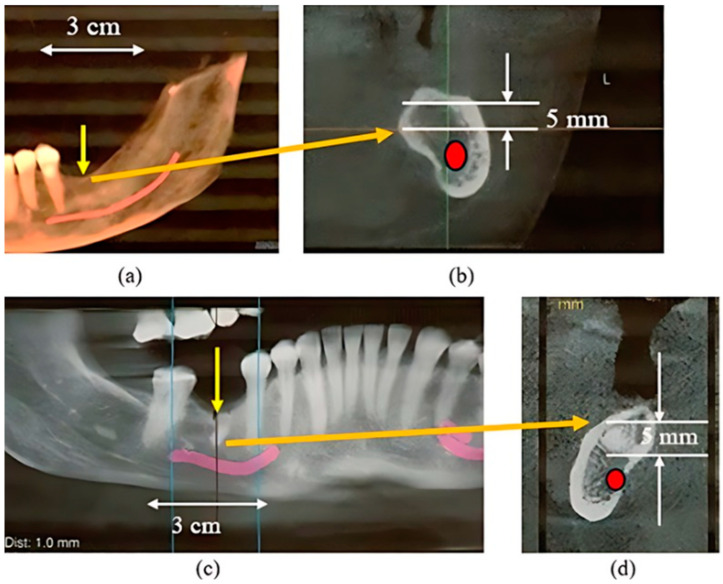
CBCT images Patients 1 (a,b), Patient 2 (c,d). (a,c): Extensive alveolar resorption at the planned sites (yellow arrows). (b,d): Cross-sectional views of the proposed positions of the planned RBIs where there was little crestal clearance (approximately 5 mm) above the inferior alveolar nerve (red).
